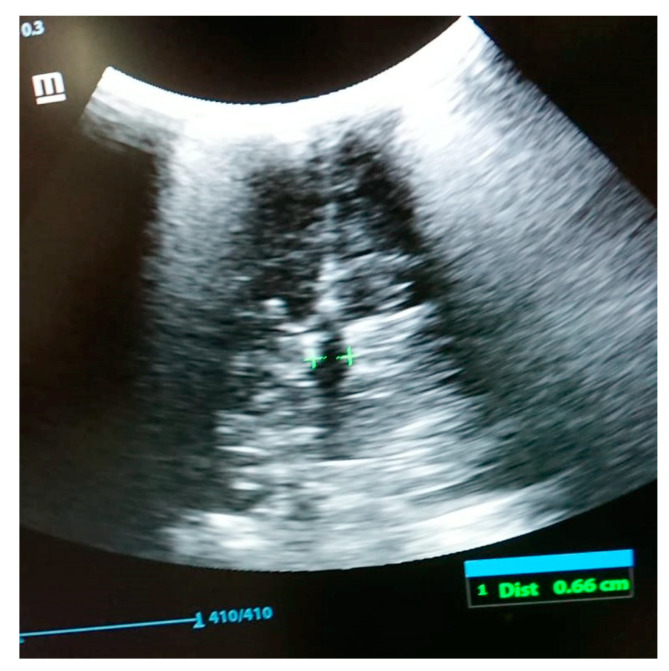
Brain ultrasound: enlargement of the third ventricle and agenesis of the corpus callosum.
